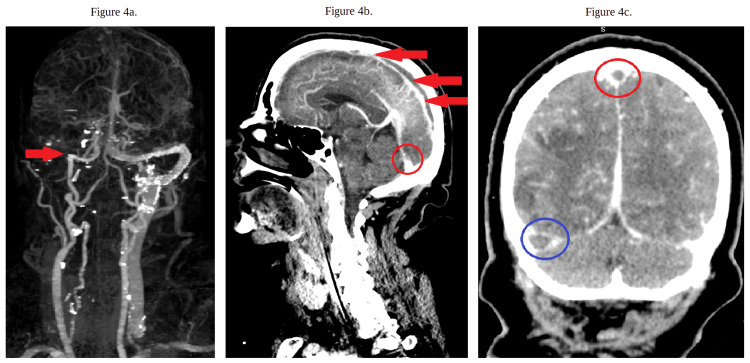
CT angiography of the brain and neck with contrast (a) The red arrow indicates a lack of flow. (b) The red circle highlights thrombosis of the confluence of sinuses, also known as the torcula. The red arrows also show superior sagittal sinus thrombosis. (c) The red circle indicates a filling triangular defect which portrays the empty delta sign which depicts dural venous sinus thrombosis of the superior sagittal sinus. The blue circle shows thrombosis of the right transverse and right sigmoid sinus. CT: computed tomography
